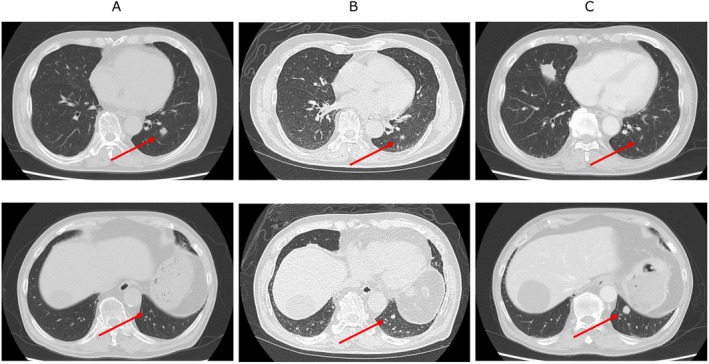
Serial chest computed tomography demonstrating heterogeneous temporal changes of multiple pulmonary nodules. Representative axial images obtained at baseline (A), 2 weeks after the initial CT and before antimicrobial therapy (B), and 8 weeks after the initial CT and after therapy initiation (C). The upper row shows spontaneous regression of one of the multiple pulmonary nodules (arrows), whereas the lower row demonstrates progressive enlargement of another distinct nodule despite antimicrobial therapy; this lesion was subsequently selected for surgical lung biopsy (arrows).
